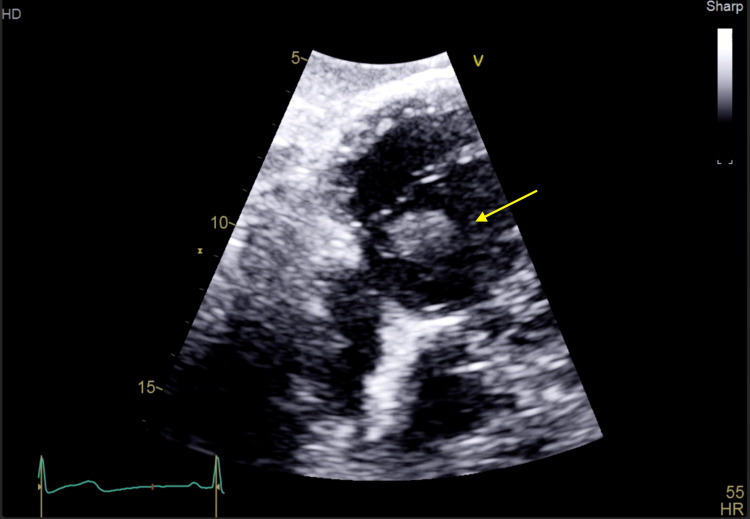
Transthoracic echocardiogram (subcostal view) demonstrating a tricuspid valve mass herniating into the right ventricular cavity. The arrow annotation demonstrates a tricuspid valve mass herniating into the right ventricular cavity.
